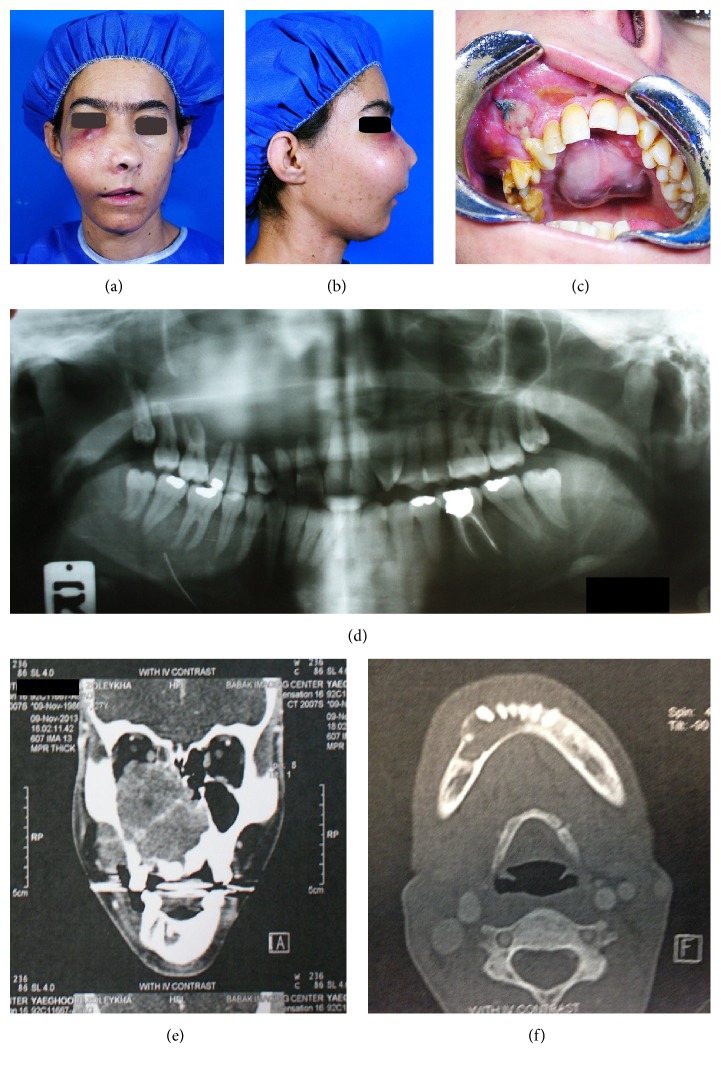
After 6 months, preoperative (a, b) extraoral frontal and lateral photography were carried out. (c) Intraoral photography. (d) Panoramic view. (e) Coronal contrast enhanced CT scan image. (f) Axial contrast enhanced CT scan image.
